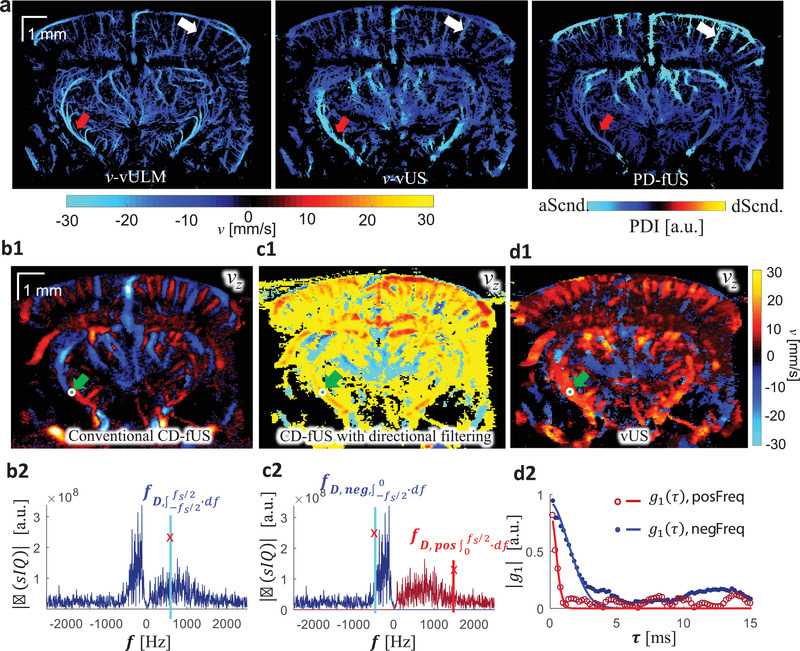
In vivo results comparison. a) In vivo ascending flow results obtained with vULM, vUS, and PD‐fUS, where vULM is used as the comparison standard and the ULM spatial mask was applied to both vUS and PD‐fUS. b1) Axial velocity (*v_z_*) map obtained with conventional CD‐fUS; b2) Doppler frequency (*f*
